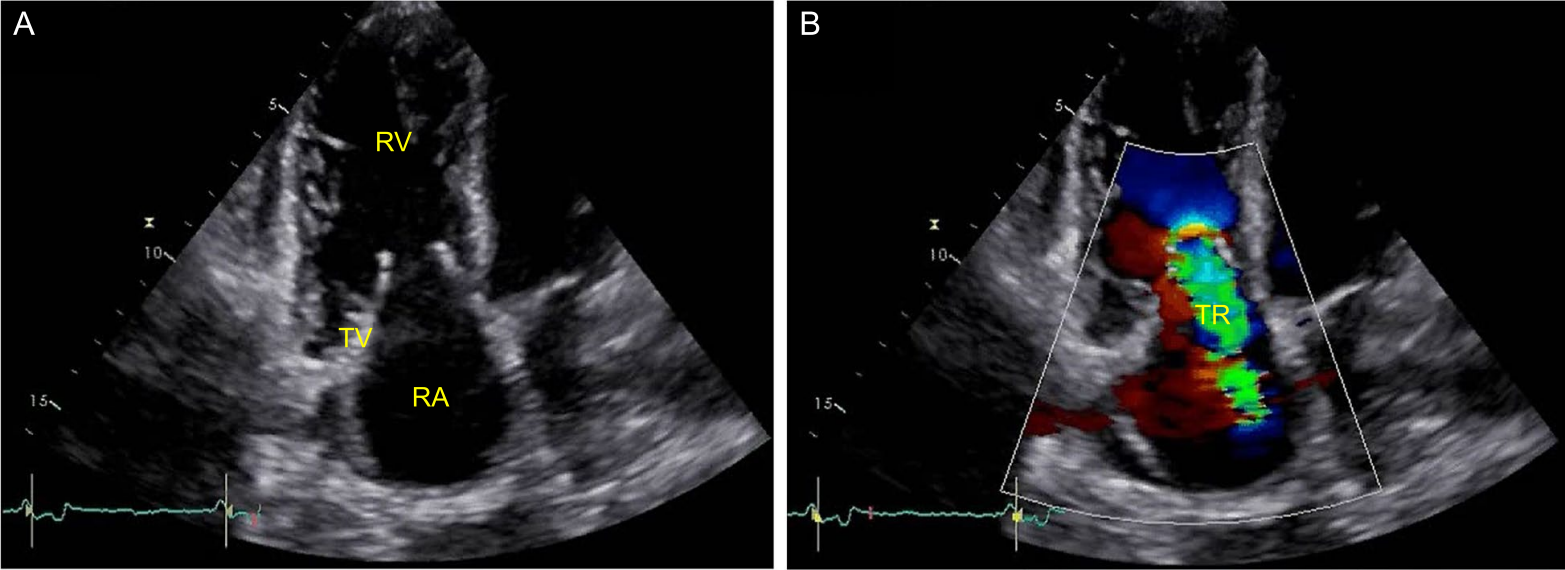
Echocardiographic imaging of the right heart with thickened, retracted, and immobile tricuspid valve leaflets (A) resulting in severe regurgitation (B) in a patient with carcinoid heart disease (apical four-chamber view), RA, right atrium; RV, right ventricle; TV, tricuspid valve; TR, tricuspid regurgitation
